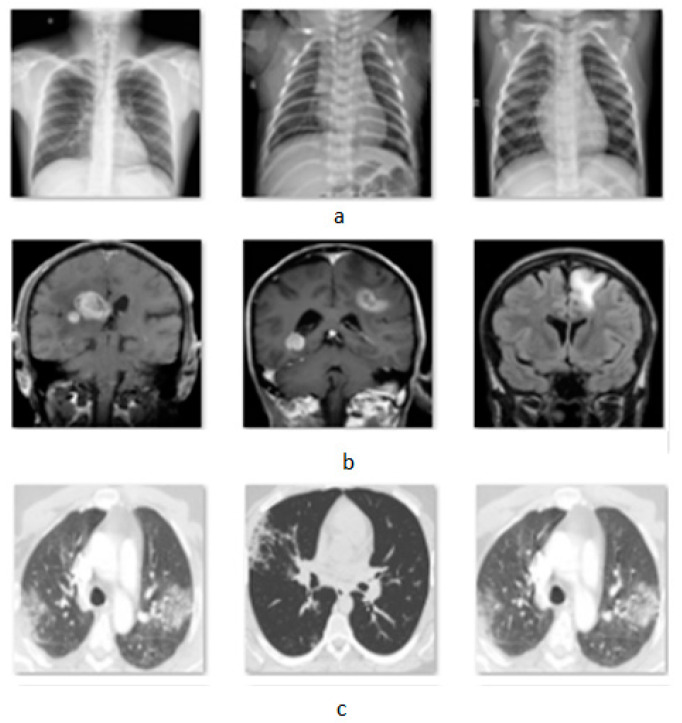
Images from three datasets, (a) X-rays, (b) MRIs, and (c) CT-Scans.
